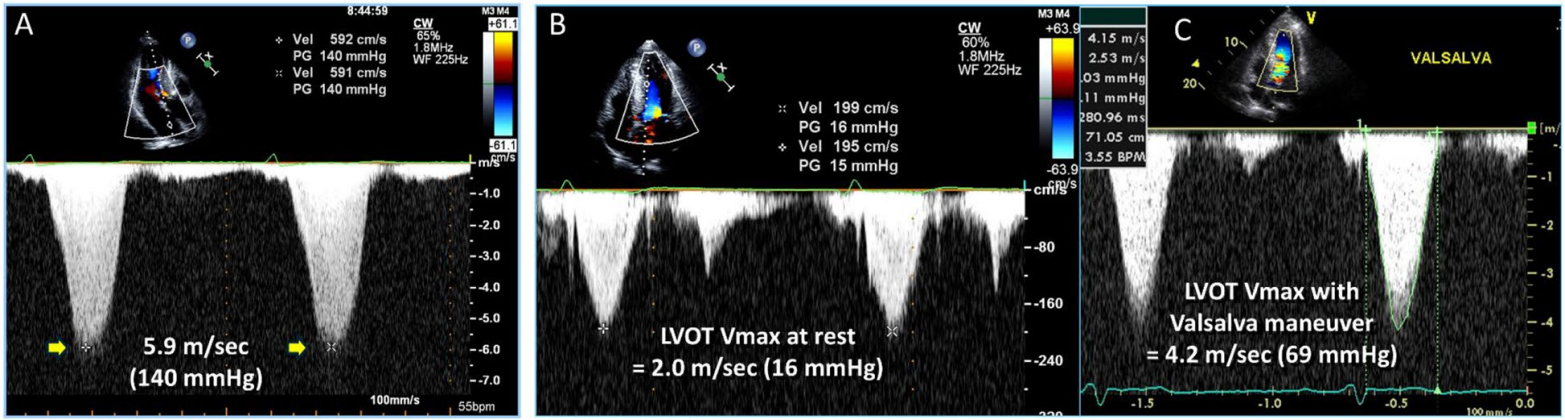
Representative Doppler tracings of resting and latent left ventricular outflow tract obstruction. A Markedly increased systolic peak velocity with a typical delayed peak and dagger shape is recorded at rest, suggestive of resting outflow tract obstruction. In some patients, (B) peak velocity at rest is not high but (C) increases significantly with the Valsalva maneuver, suggesting latent outflow tract obstruction
